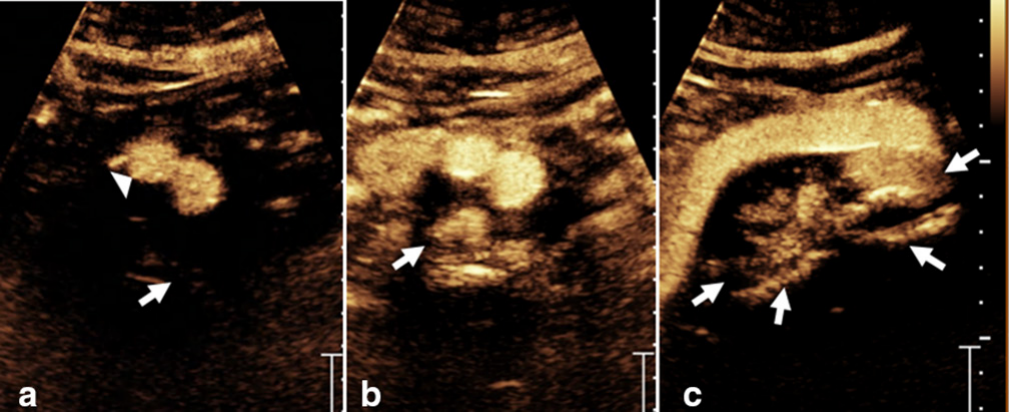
Transverse (a, b) and sagittal (c) post-contrast ultrasound images show a faint linear enhancing vessel outside the aneurysm sac (A, arrow) and contrast within the stent (a, arrowhead) obtained at 22 s after injection with progressively increased contrast in the aneurysm sac (b, arrow) with time. The filling of the aneurysmal sac and lumbar vessels are better seen on the sagittal image (c, arrows). The findings are compatible with persistent Type II endoleak.
